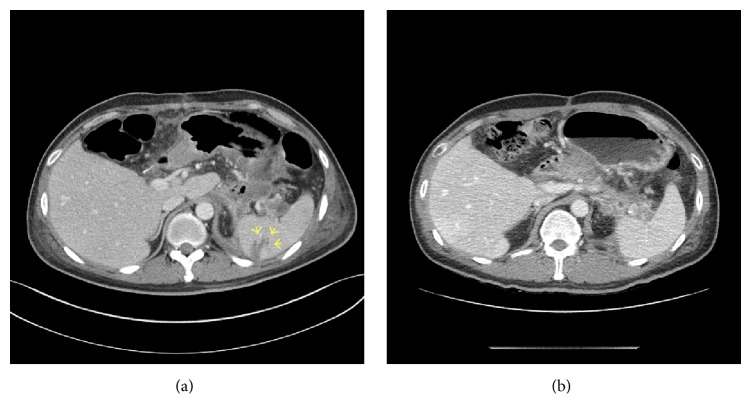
(a) CT scan on 2 days following the SAE. A small area of splenic infarction was seen. (b) CT scan on 4 months following the SAE. The infarcted area was not observed any more.
